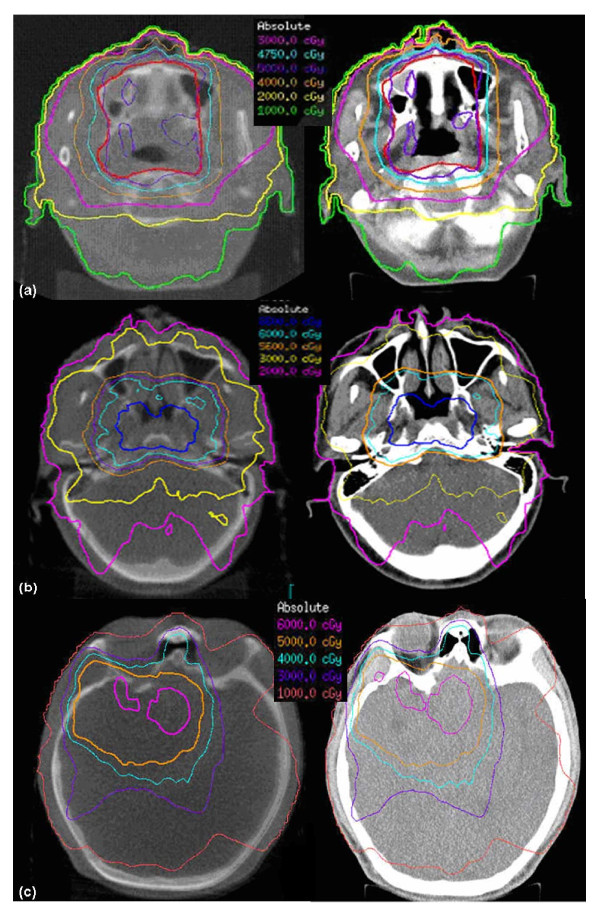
Dose distributions of three clinical cases. The transverse views of dose distributions of the NK/T lymphoma (a), NPC (b) and brain tumor (c). Left: calculated dose based on CBCT images; Right: calculated dose based on planning CT images. They show good agreement on both relative high and low isodoses.
